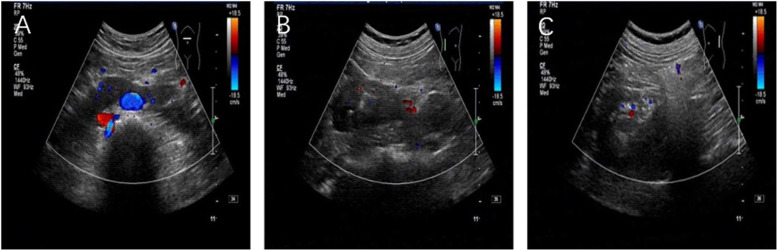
B-mode ultrasonography. A The ultrasound probe is placed on the midline of the upper abdomen of the umbilical cord. B The ultrasound probe is placed on the right abdomen. C The ultrasound probe is placed on the left abdomen
